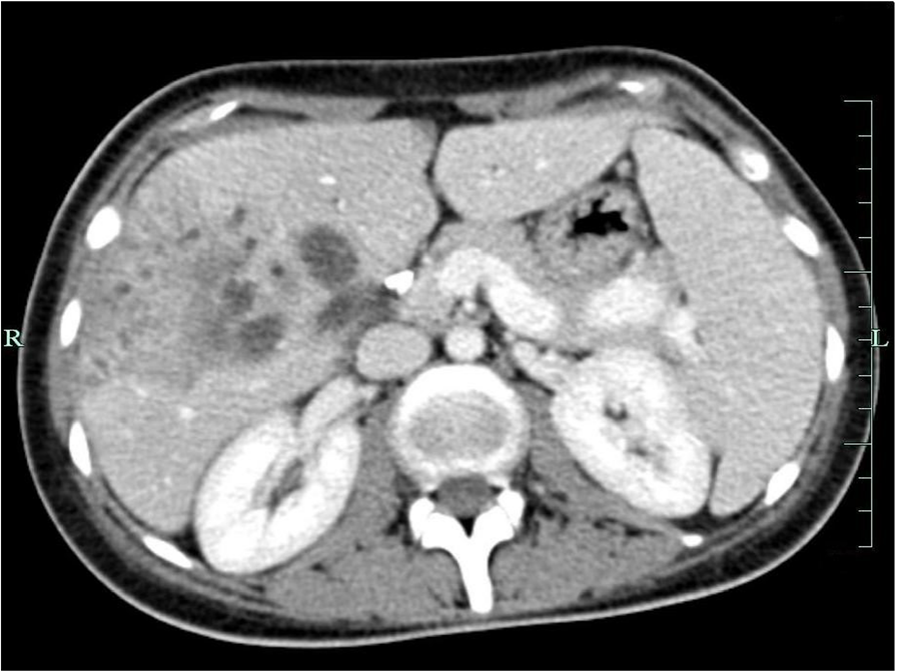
Computed tomography (CT) scan showing right liver lobe ischemia and abscess 2 months following laparoscopic cholecystectomy with combined bile duct and right hepatic artery injury
